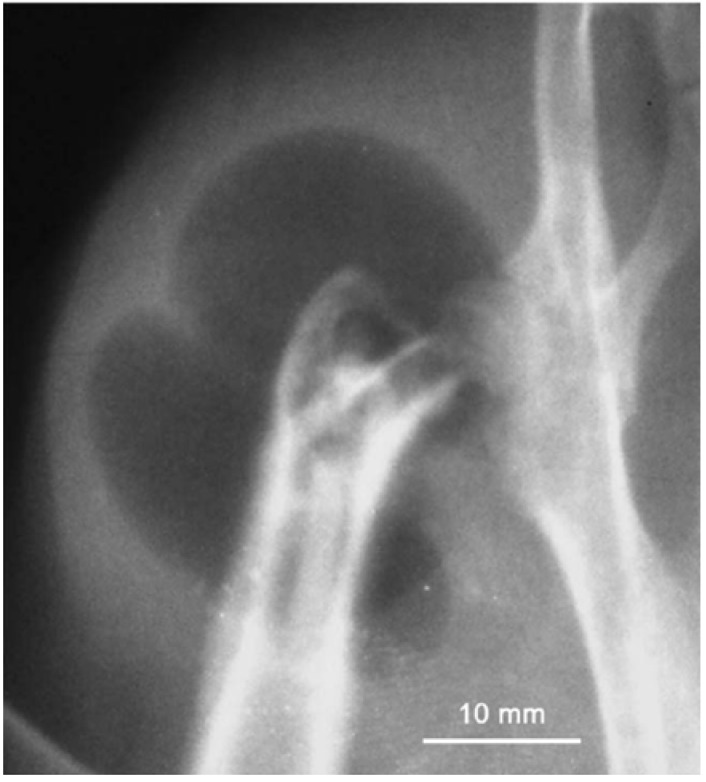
Subcutaneous gas bubble observed on postoperative radiographs for 4 weeks during magnesium implant degradation [68].
